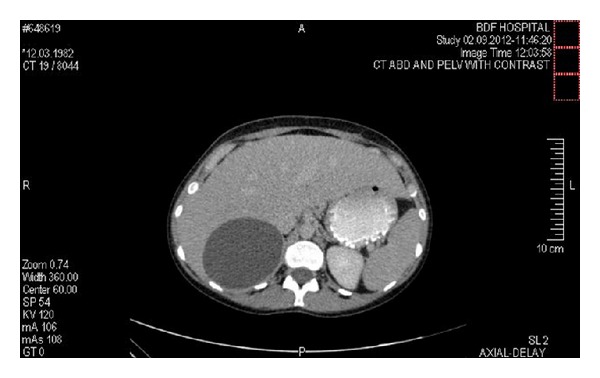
CT abdomen and pelvis showing the cyst.
